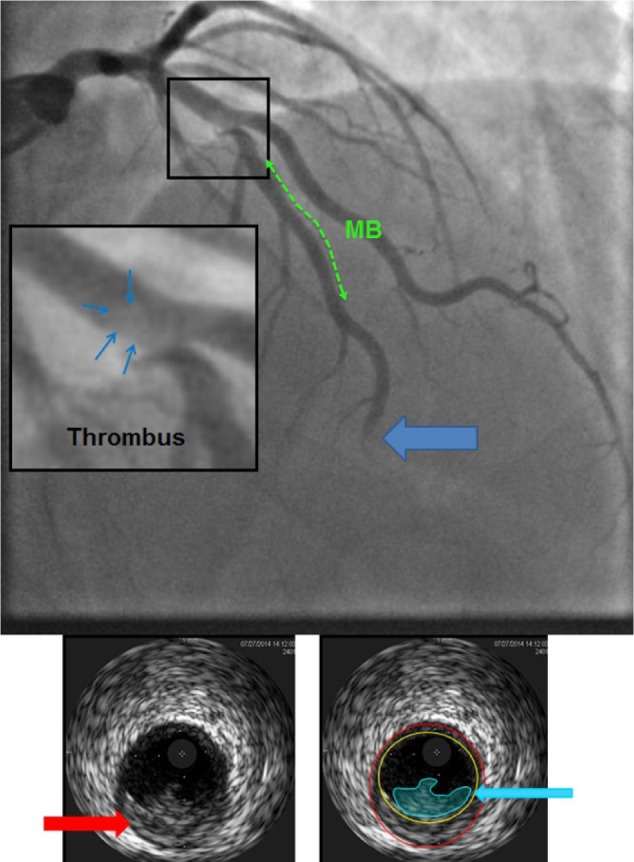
Coronary angiogram showing a hazy lesion in the proximal LAD, consistent with an acute thrombus formation. The green area shows the MB segment. The large blue arrow points to the total occlusion of the mid to distal LAD from distal embolization. On the left: a magnification of the hazy area in the LAD, consistent with a thrombus. Bottom images: the left picture shows an IVUS image of the ruptured plaque (red arrow) and to the right the thrombus on top of the plaque (blue arrow). MB, myocardial bridge; LAD, left anterior descending artery; IVUS, intravascular ultrasound.
